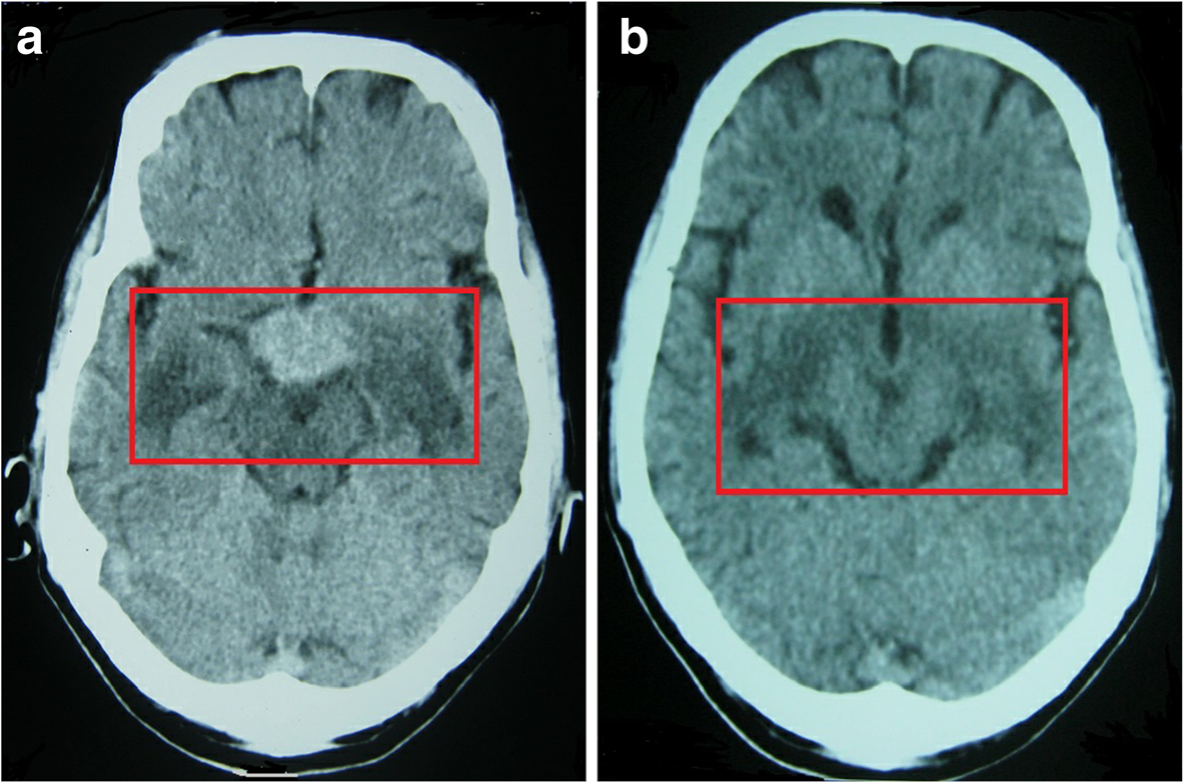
a Non contrast CT images. Transverse section of the brain at the thalamic level. This image showed a high density mass-like lesion in the hypothalamus region with the surrounding hypodense area in the brain. This lesion was initially reported as a mass lesion/ hemorrhage with surrounding edema. b Non contrast CT images. Transverse section of the brain at upper brain stem level
